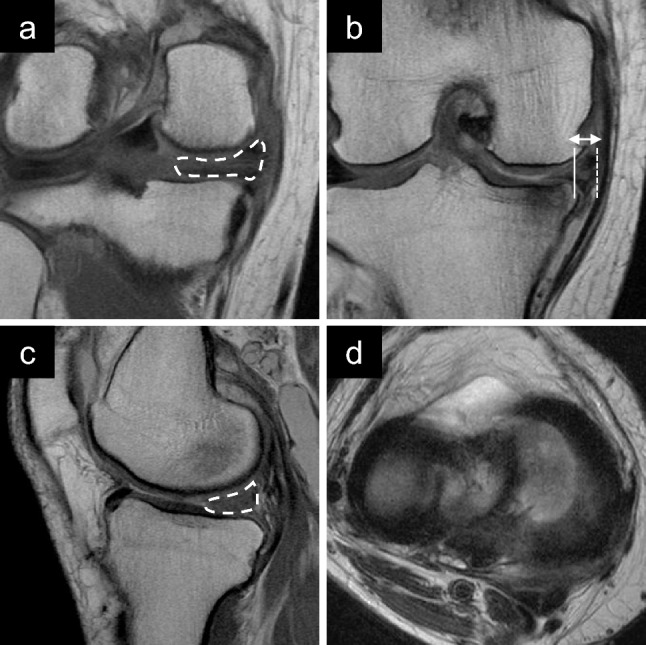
Preoperative magnetic resonance images of type 4 medial meniscus (MM) posterior root tears in the right knee. a Coronal view of the MM posterior portion showing a giraffe neck sign (dashed area). b Coronal view of the middle portion of the MM showing a medial extrusion (double arrow). c Sagittal view showing a ghost sign (dotted area). d Axial view showing no radial tears adjacent to the posterior root of the MM
